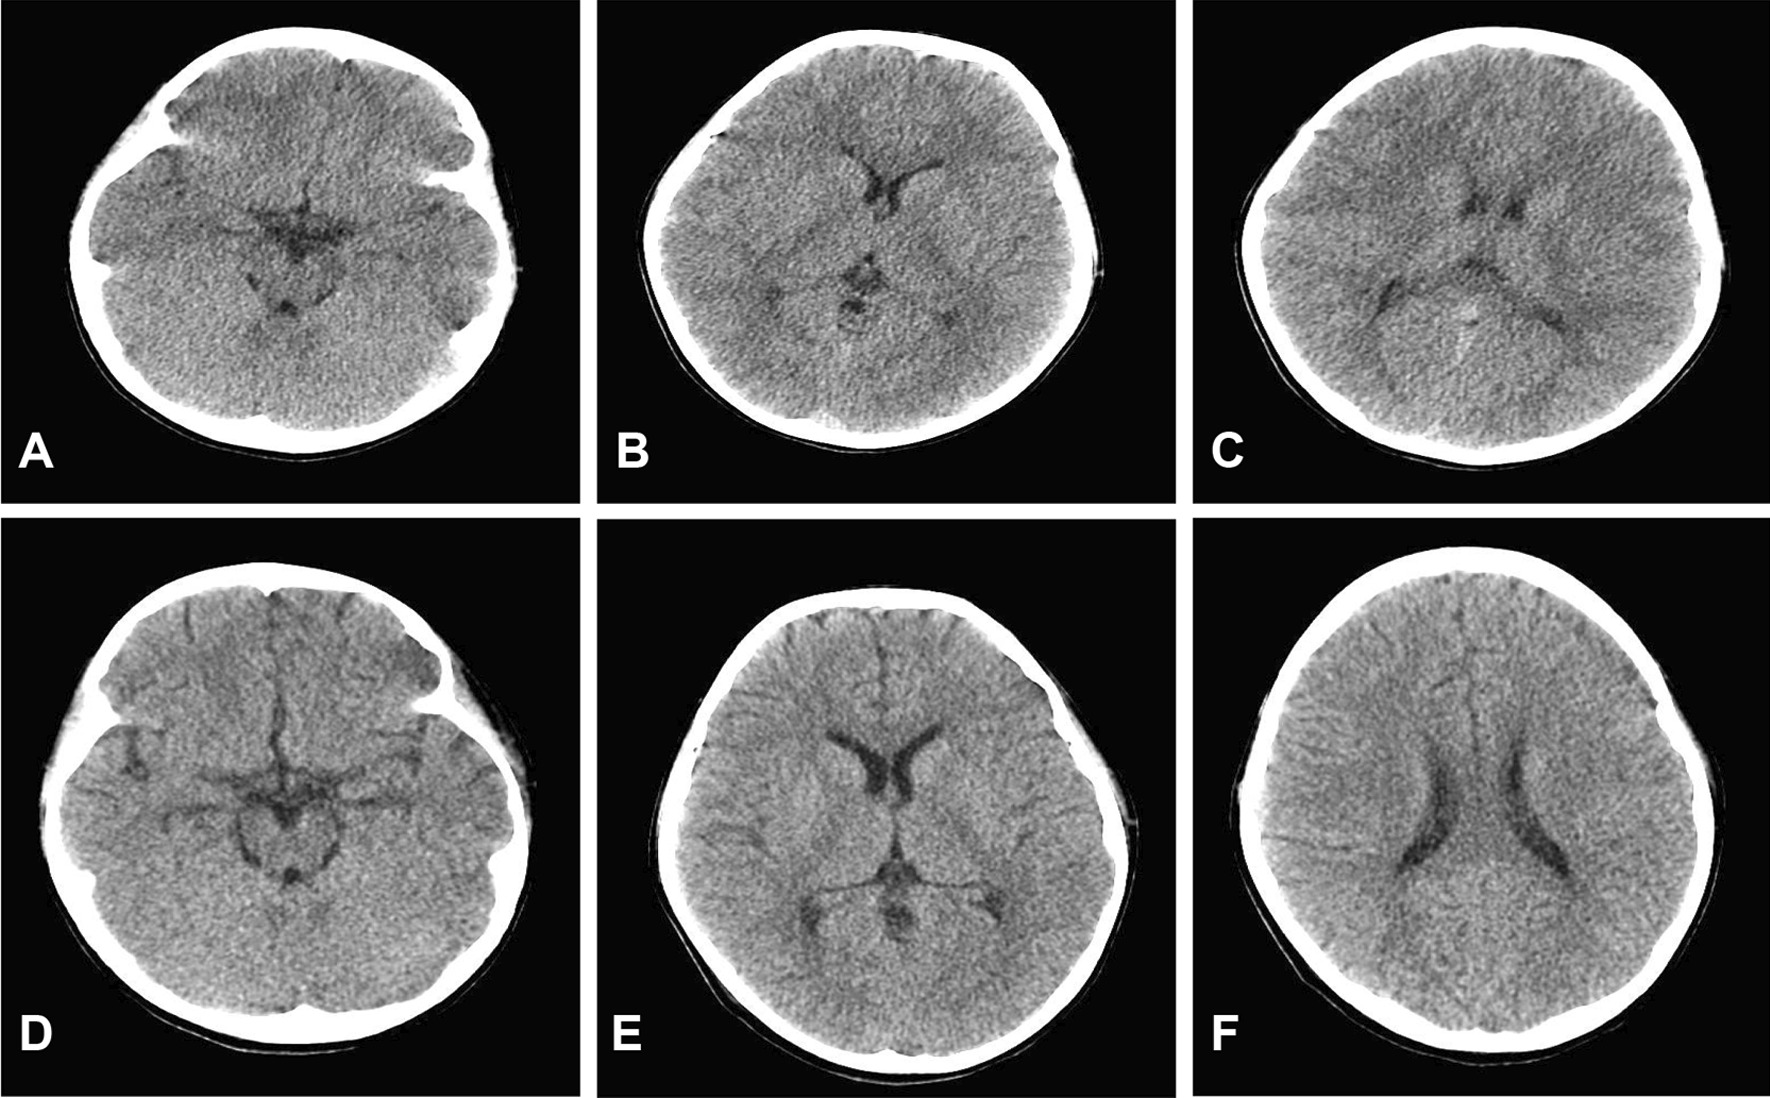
Cerebral computed tomography results. A–C Images obtained on admission Day 1 show a finding suggestive of diffuse encephaledema. D–F Images obtained on admission Day 7 show improvement of encephaledema
